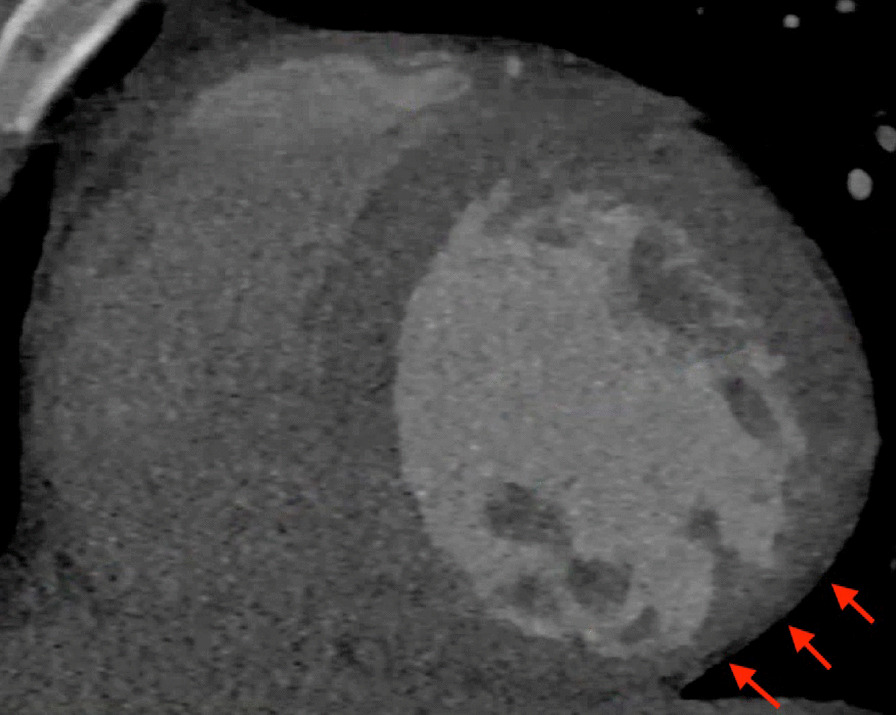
Case 6. Figure 3. CT coronary angiogram. LV inferolateral myocardial wall thinning (red arrows) present
